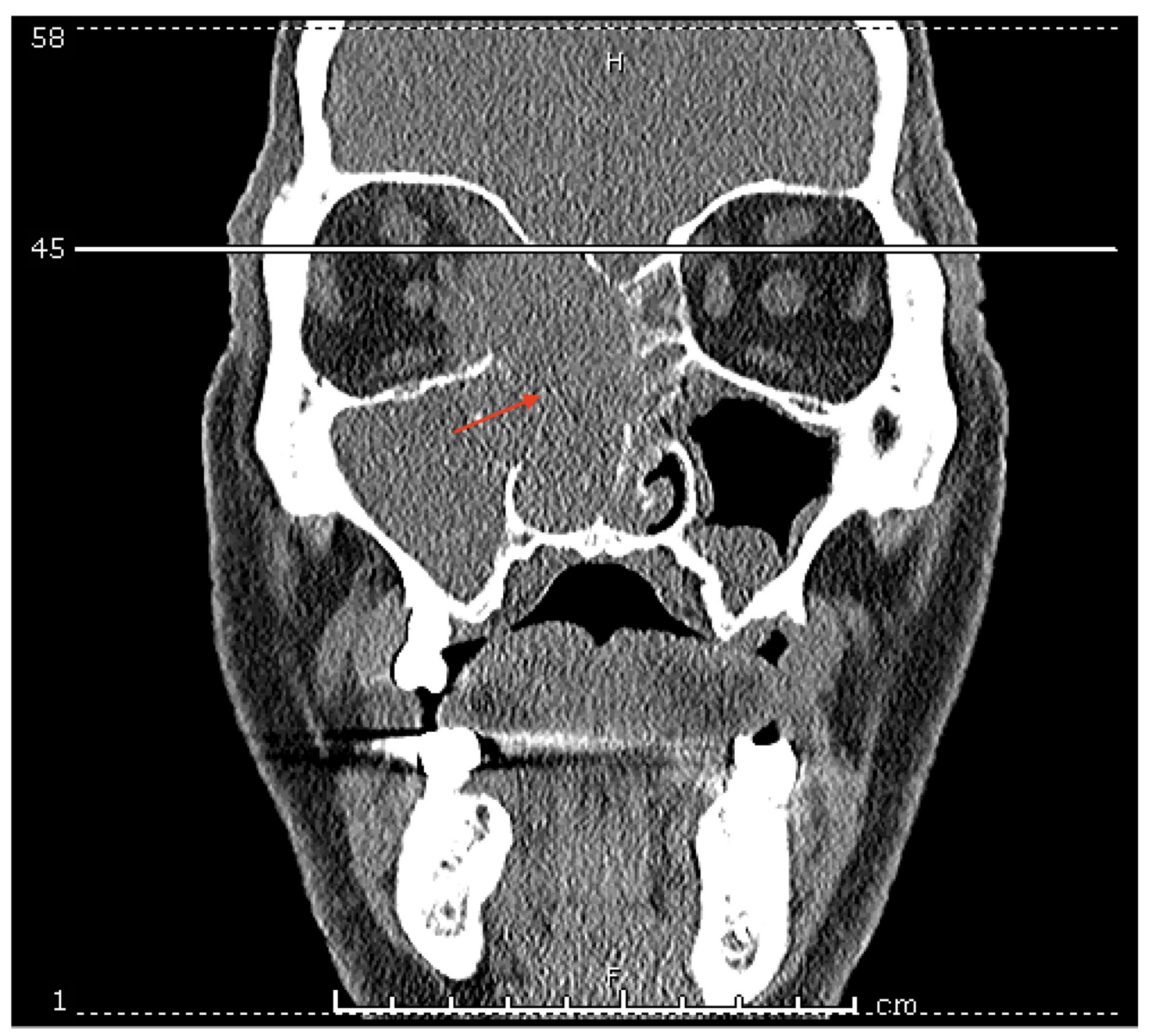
Maxillofacial CT. Red arrow shows 3.5 cm x 1.5 cm mass which has completely obstructed the right nasal cavity and eroded into the nasal septum along with leftward deviation of the septum.
